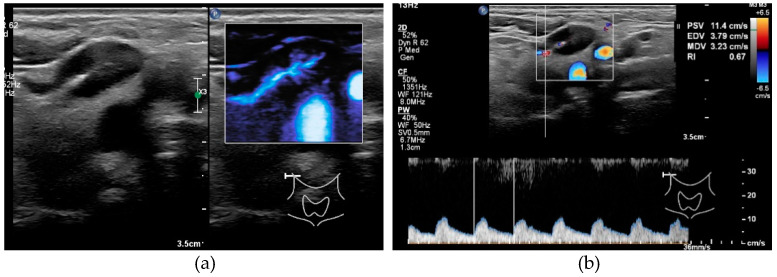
Ultrasound features of a benign node. (a) Hilum sign in a benign node, no peripheral vascularity. (b) Measurement RI 0.67.
